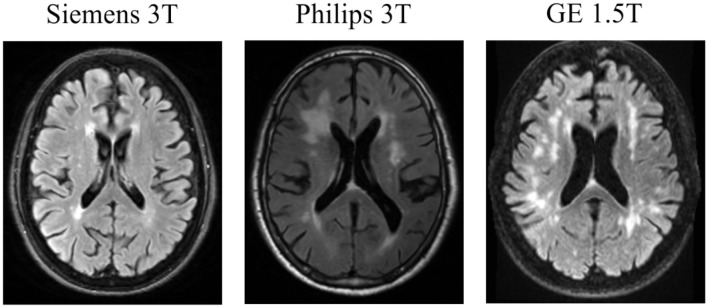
Comparison of MRI scans between different domains.
